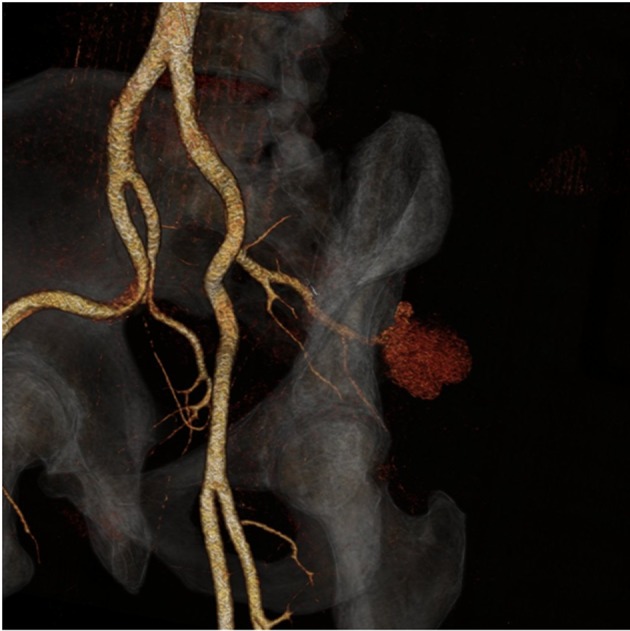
A 3D reformat of the contrast-enhanced computed tomography of the pelvis showing a ruptured aneurysm with an associated pseudoaneurysm.
